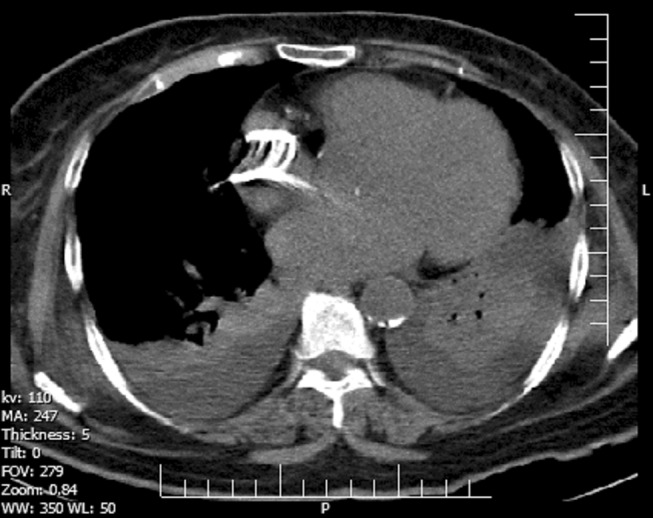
The mediastinal window in the spiral chest computed tomography scan shows a high‐density focal point with a streaky artifact in the right atrium, consistent with cement embolization.
